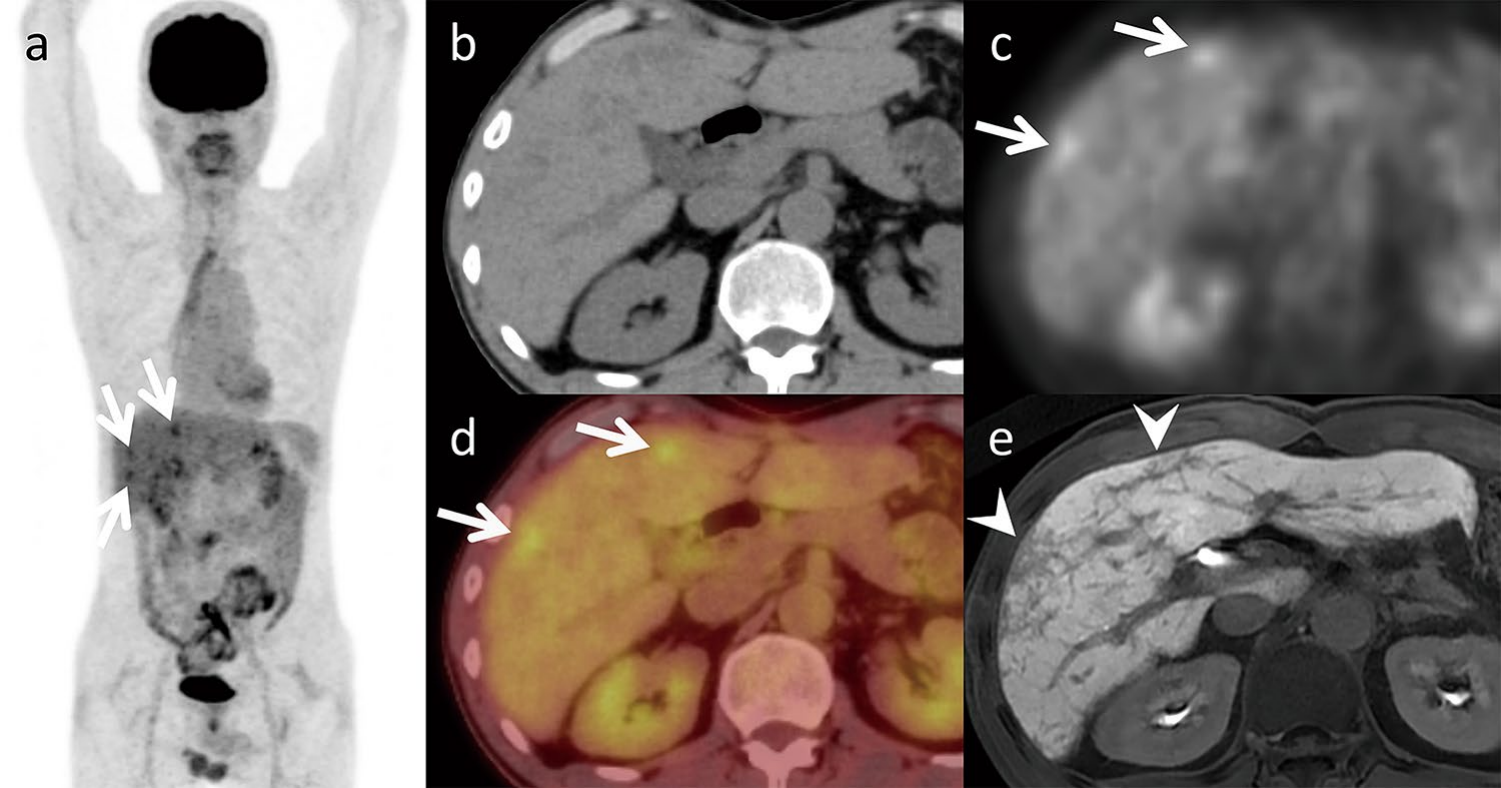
Sinusoidal obstruction syndrome (SOS) in an asymptomatic 52-year-old man, who was undergoing chemotherapy (S-1) for esophageal cancer. Fluorodeoxyglucose positron emission tomography (FDG-PET) (a maximum intensity projection of FDG-PET, b computed tomography (CT), c FDG-PET image, and d fused FDG-PET/CT image) demonstrates several focal tracer uptakes (maximum standardized uptake value, 4.0–5.0) in the liver (arrows). Multiple liver metastases were suspected; however, the hepatobiliary phase demonstrates reticular hypo-intensity in the liver (arrowheads), typical of SOS
